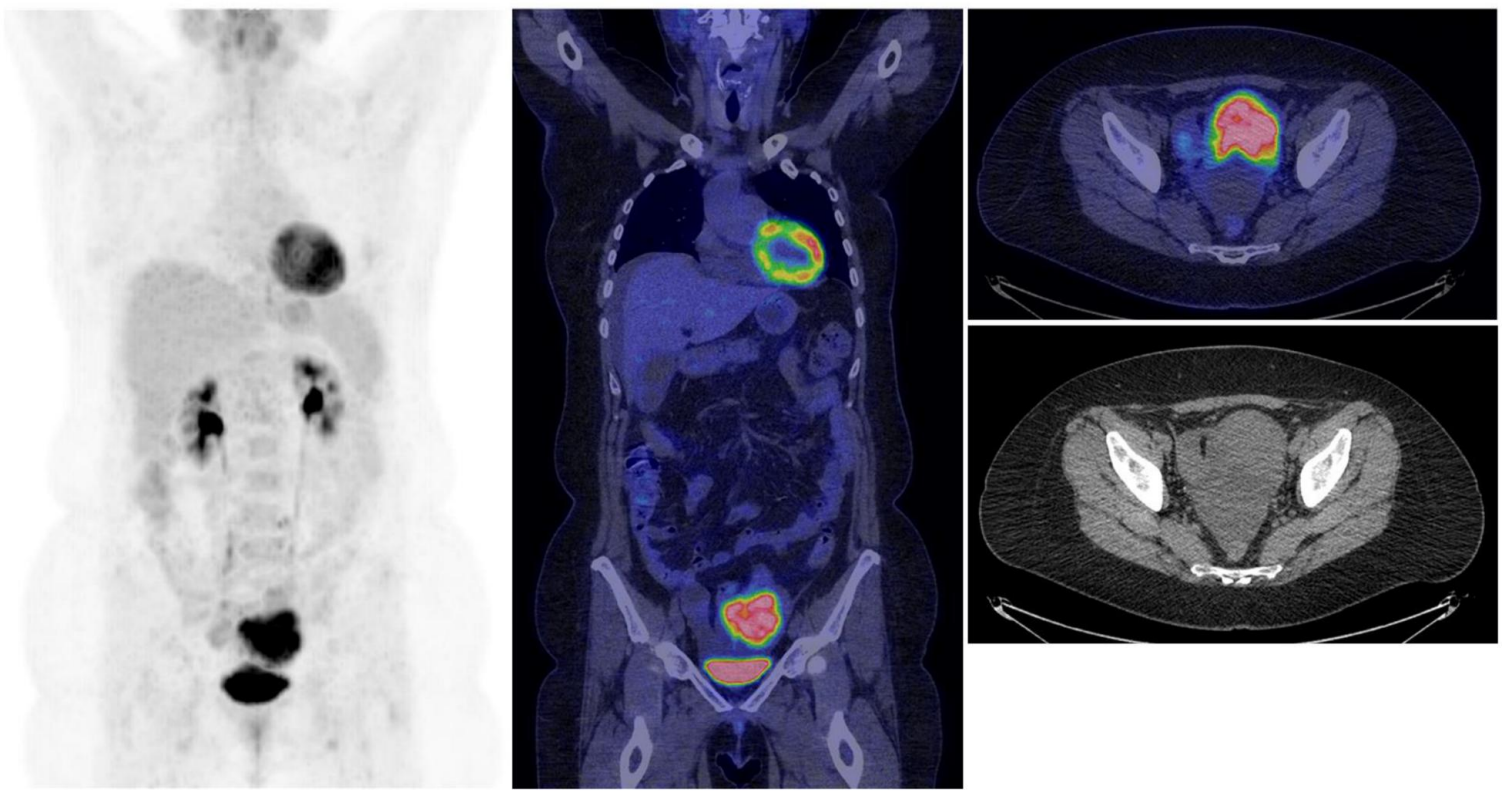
A 55-year-old woman was admitted with acute abdominal pain and examined via a CT scan showing an enlarged ovary; thereafter, the patient was referred for an ^18^F-fluorodeoxyglucose PET CT (FDG PET/CT) scan. The FDG PET/CT scan revealed high FDG-uptake in a suspected primary ovarian tumour originating from the left ovary with both solid and cystic components with no evidence of disseminated disease. The patient had previously been treated for breast cancer by lumpectomy and axillary lymph node dissection, followed by chemotherapy, radiation therapy and anti-estrogen therapy six and a half years prior to the current FDG PET/CT and the pathological diagnosis of the ovarian tumour was metastasis from the previous breast cancer. The SUV_max_ of the metastasis was 14.3.
